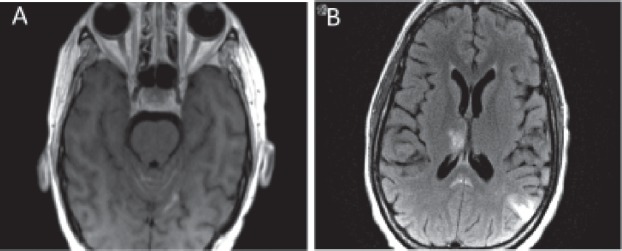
MRI brain showing leptomeningeal disease. (a) T1 axial MRI with contrast depicting new enhancement along superior cerebellar folia compatible with leptomeningeal disease. (b) Abnormal leptomeningeal enhancement along posterior margin of splenium of corpus callosum.
